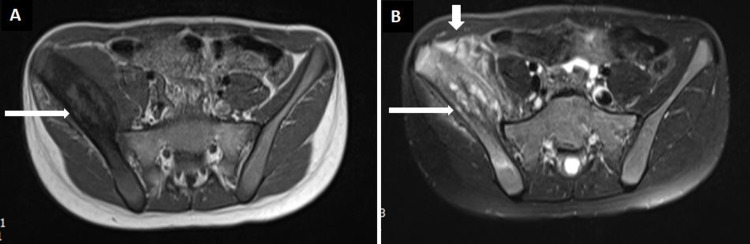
MRI of pelvis. A: T1-Weighted axial section demonstrating right iliac bone and surrounding soft tissue expansion and abnormal signals. B: T2-Weighted axial section at the same level showing heterogeneous high signals in the right iliac bone and surrounding soft tissue expansion and edema.
